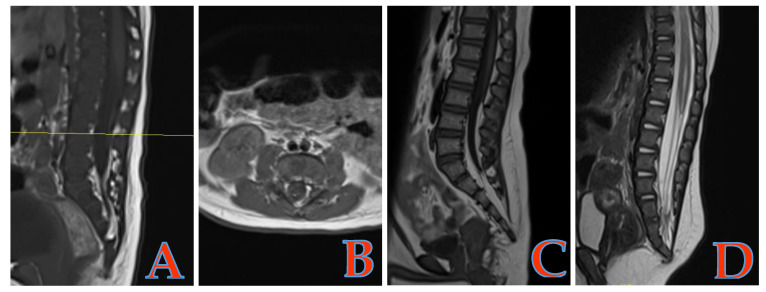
Type IV SL: The conus medullaris is in a normal position, and there is partial fatty degeneration of the filum terminale, with punctate high signals appearing on the axial T1WI. (A,B) Type III SL: The lipoma is located below the conus medullaris, merging with the subcutaneous fat through the sacral hiatus, and the entire length of the filum terminale has undergone fatty degeneration. (C) The conus medullaris has experienced dilation of the central canal. (D) SL, spinal lipomas; T1WI, T1-weighted images.
